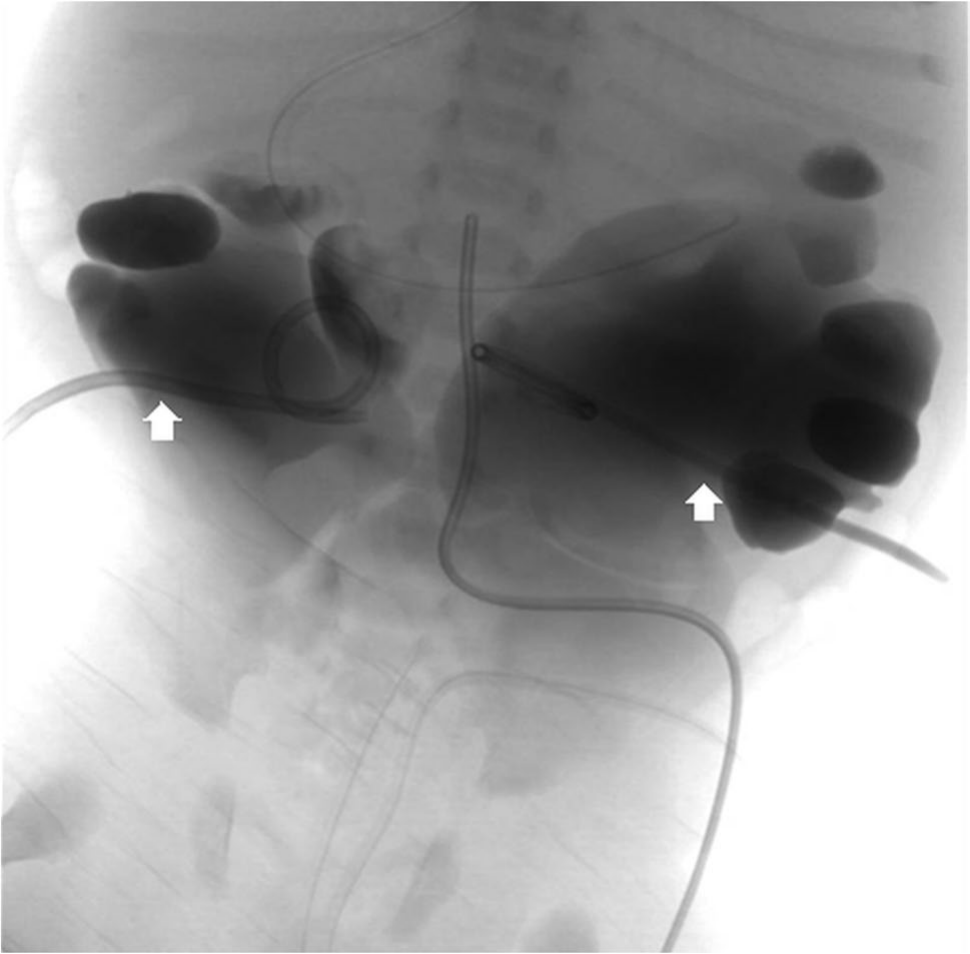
Severe congenital stenotic megaureters causing acute renal failure in a male neonate (different patient from Fig. 6). He underwent a double nephrostomy. Anteroposterior radiograph with contrast agent administered through nephrostomy tubes (*arrows*) shows severe dilatation of the urinary tracts. Case courtesy of Prof. Philippe Petit, Marseille, France
